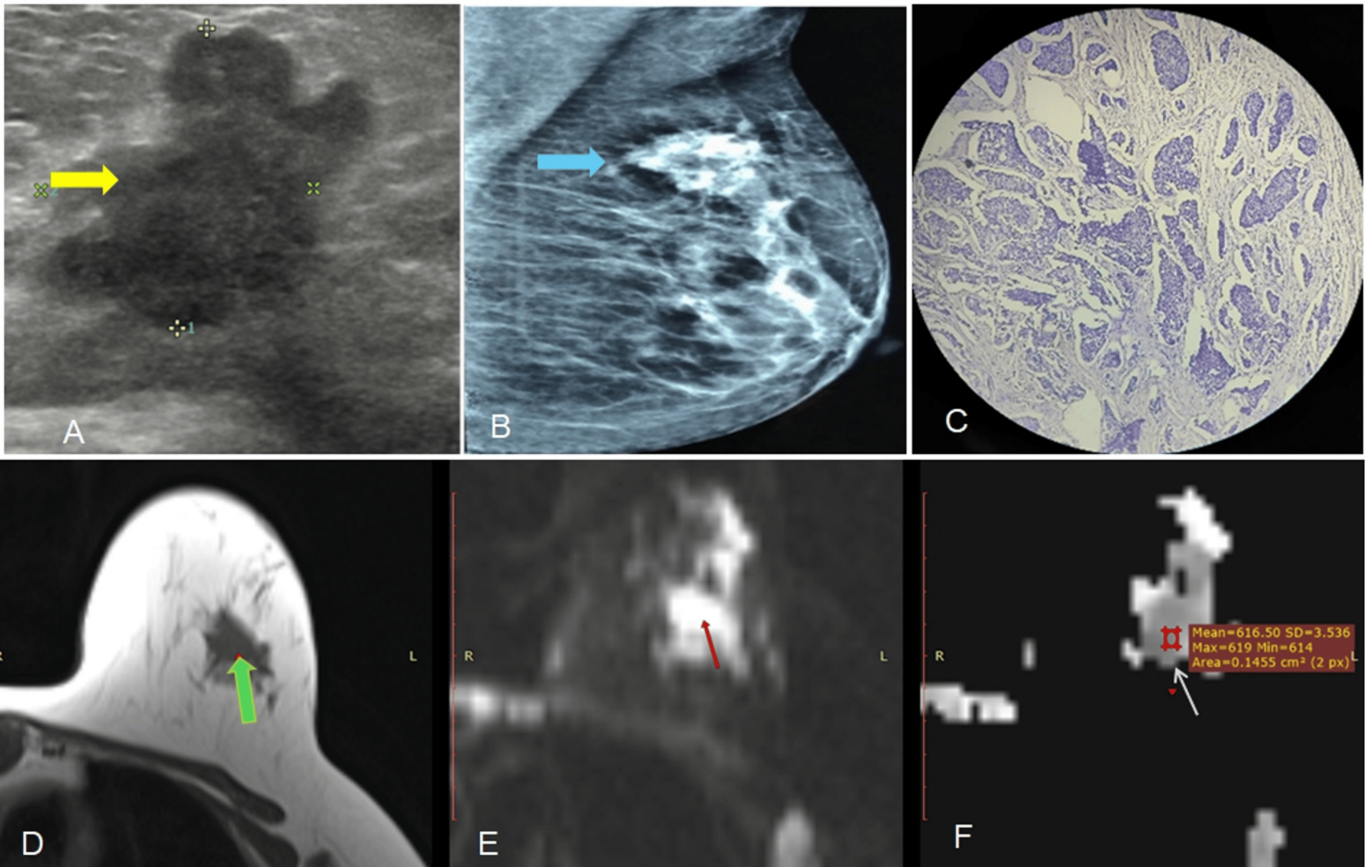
Invasive Ductal Carcinoma (A) Ultrasound demonstrates a hypoechoic, irregular lesion (yellow arrow) with microlobulated margins and non-parallel orientation, categorized as BI-RADS V.
